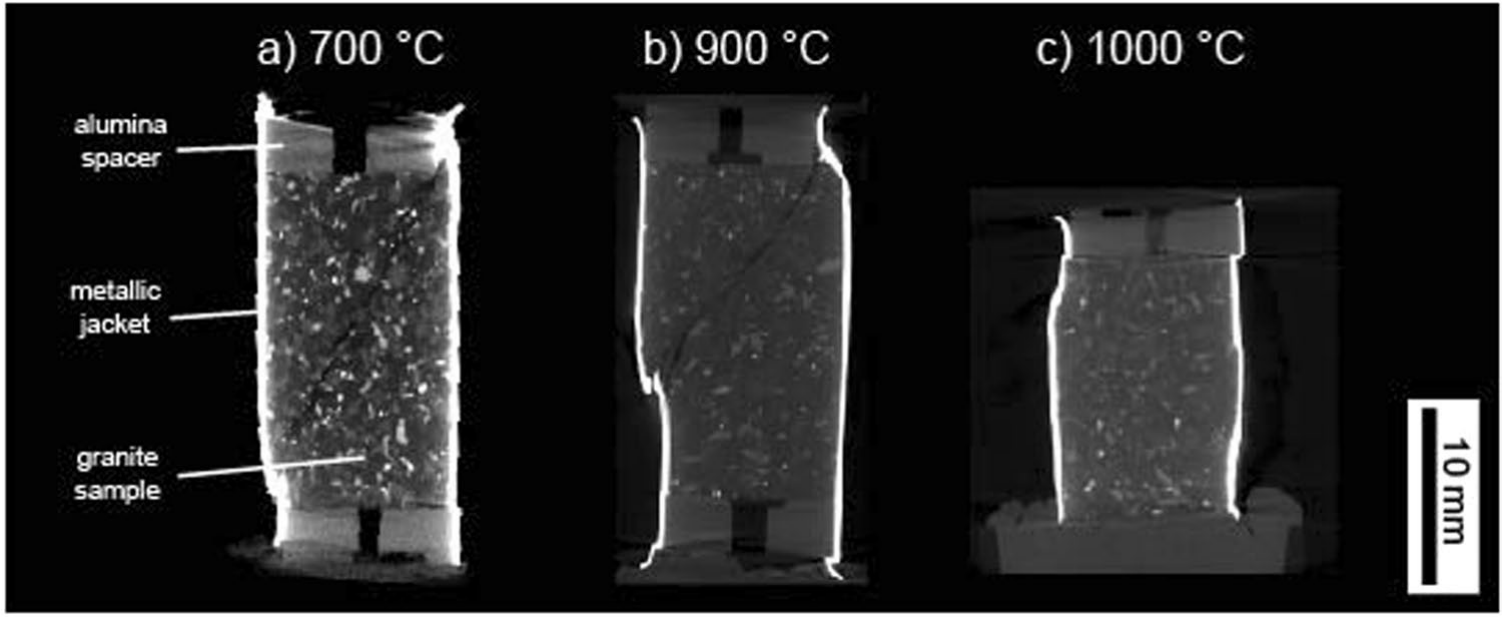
X-ray computed tomographic images of the samples of Westerly granite (thermally-shocked to 450 °C) deformed at an effective confining pressure of 100 MPa and an axial strain rate of 10^−5^ s^−1^ (the deformation experiments shown in Fig. 2). (a) Sample deformed at 700 °C. (b) Sample deformed at 900 °C. (c) Sample deformed at 1000 °C.
